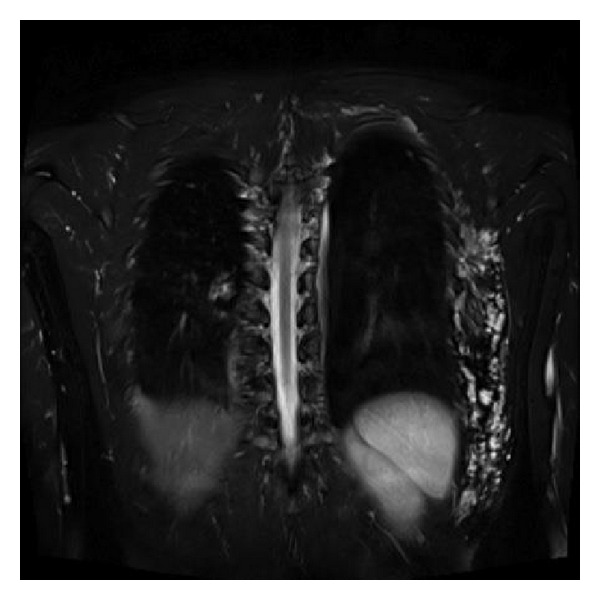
MRI scan (coronal view) of the lesion, which is located under the left scapula and extending posterolaterally down to the rim of the left 10th rib.
